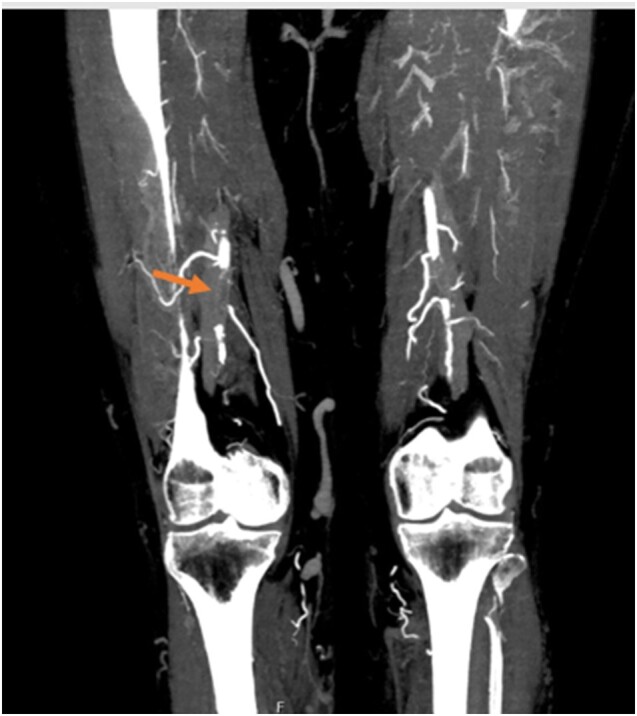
Post-procedural computed tomography angiography revealing a right deep femoral artery thrombus with distal permeabilization.
